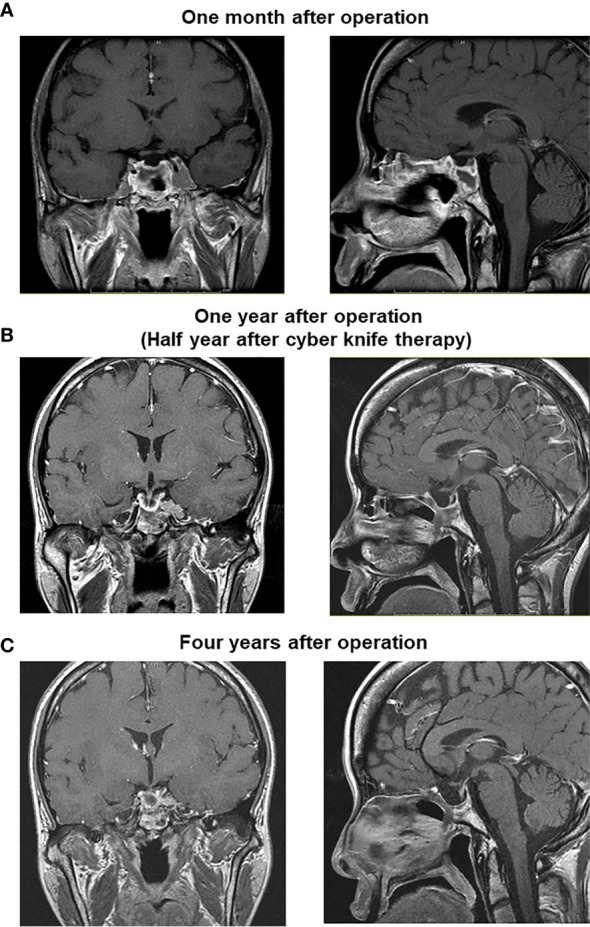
There were no particular abnormalities in brain MRI taken 1 month after operation (A), 1 year after operation (half year after cyber knife therapy) (B) and 4 years after operation (C).
